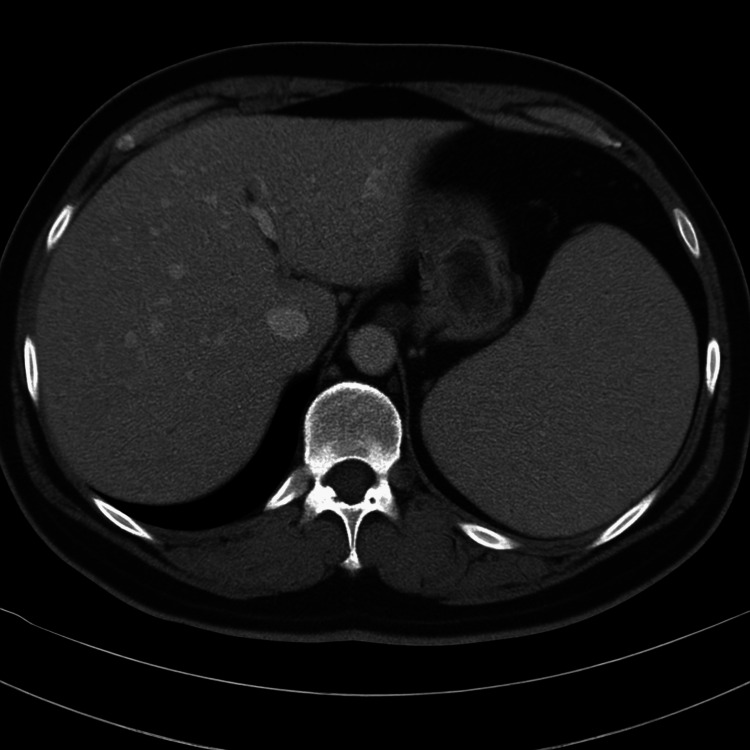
Post-procedure contrast-enhanced axial CT image showing resolution of splenic artery–splenic vein arterioportal fistula with homogeneous enhancement of the spleen and no evidence of infarction
